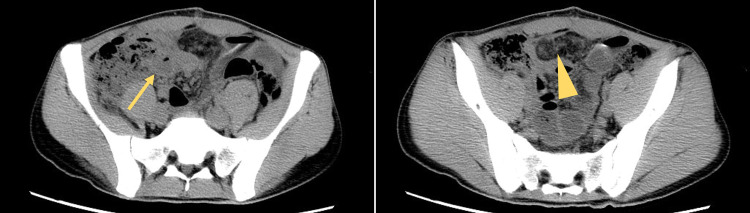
Computed tomography revealed ascites (arrow) and panniculitis (arrowhead) in the pelvic cavity, and an abscess was suspected.
